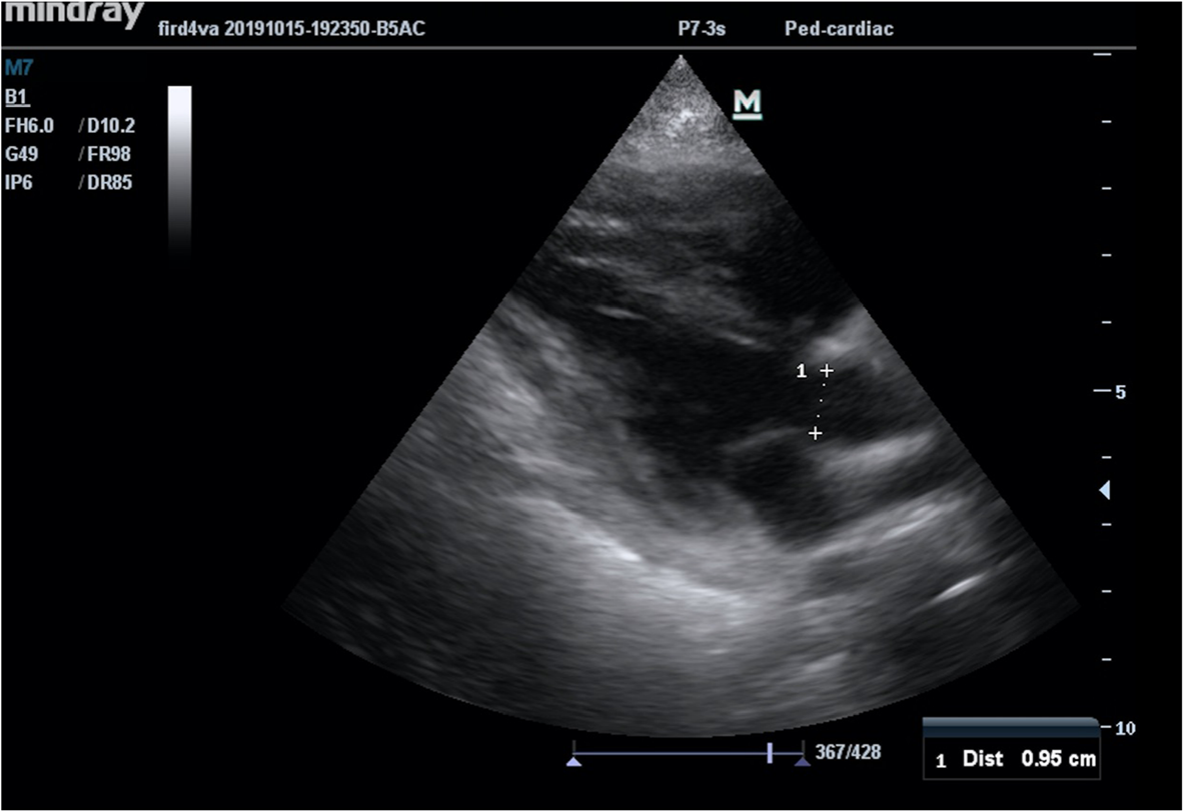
Measurement of the distance of LVOT in the parasternal longer axis
